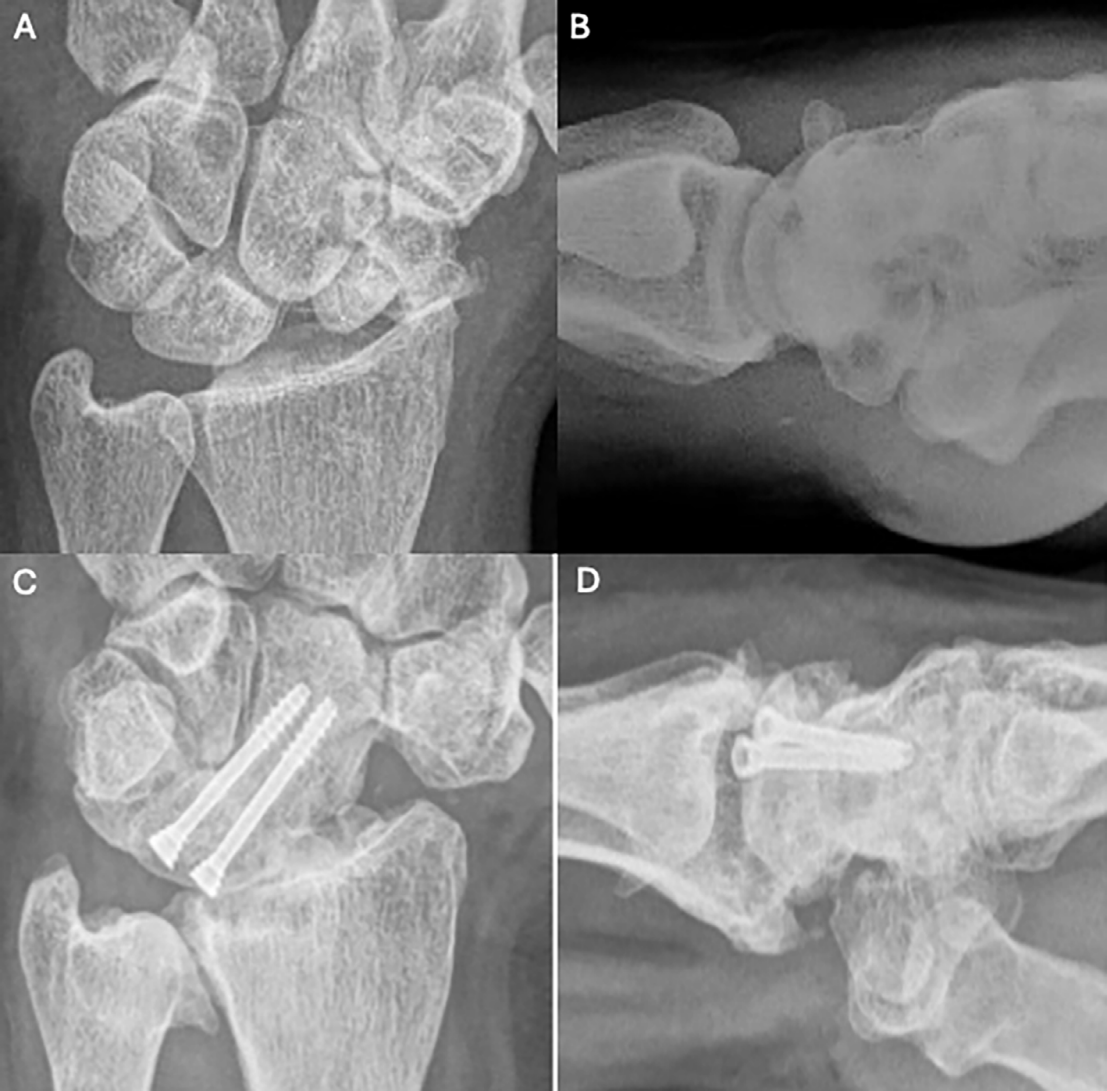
Clinical case of Capitolunate Fusion. A and B. Preoperative wrist X-rays of SNAC stage II (AP and Lateral views). C and D. Postoperative wrist X-rays (AP and Lateral views) after 4.7 years. Source: Dr. Emmanouil Apergis.
